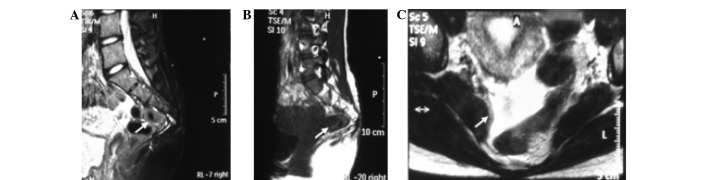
Magnetic resonance imaging (MRI) of the pelvis. (A) The mass (arrow) was detected under the sacrum (T1WI). (B) The mass (arrow) in a T2WI. (C) The mass at the right side of the rectum and with fat-like and soft tissue components. WI, weighted image.
